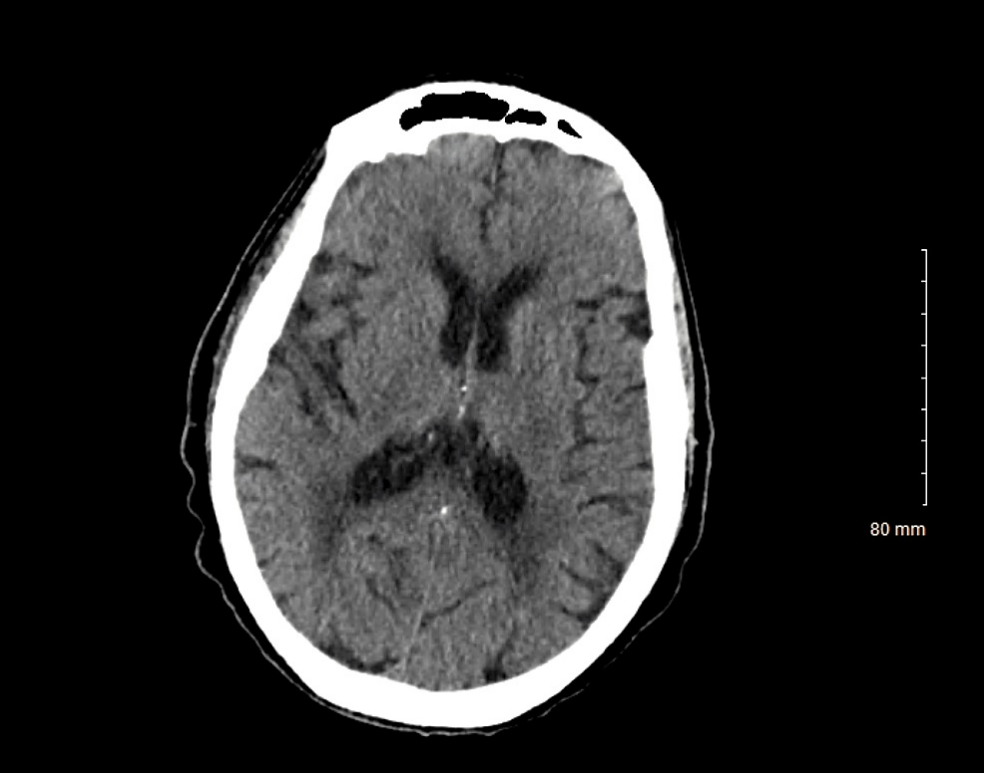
CT scan of the head without contrast.
